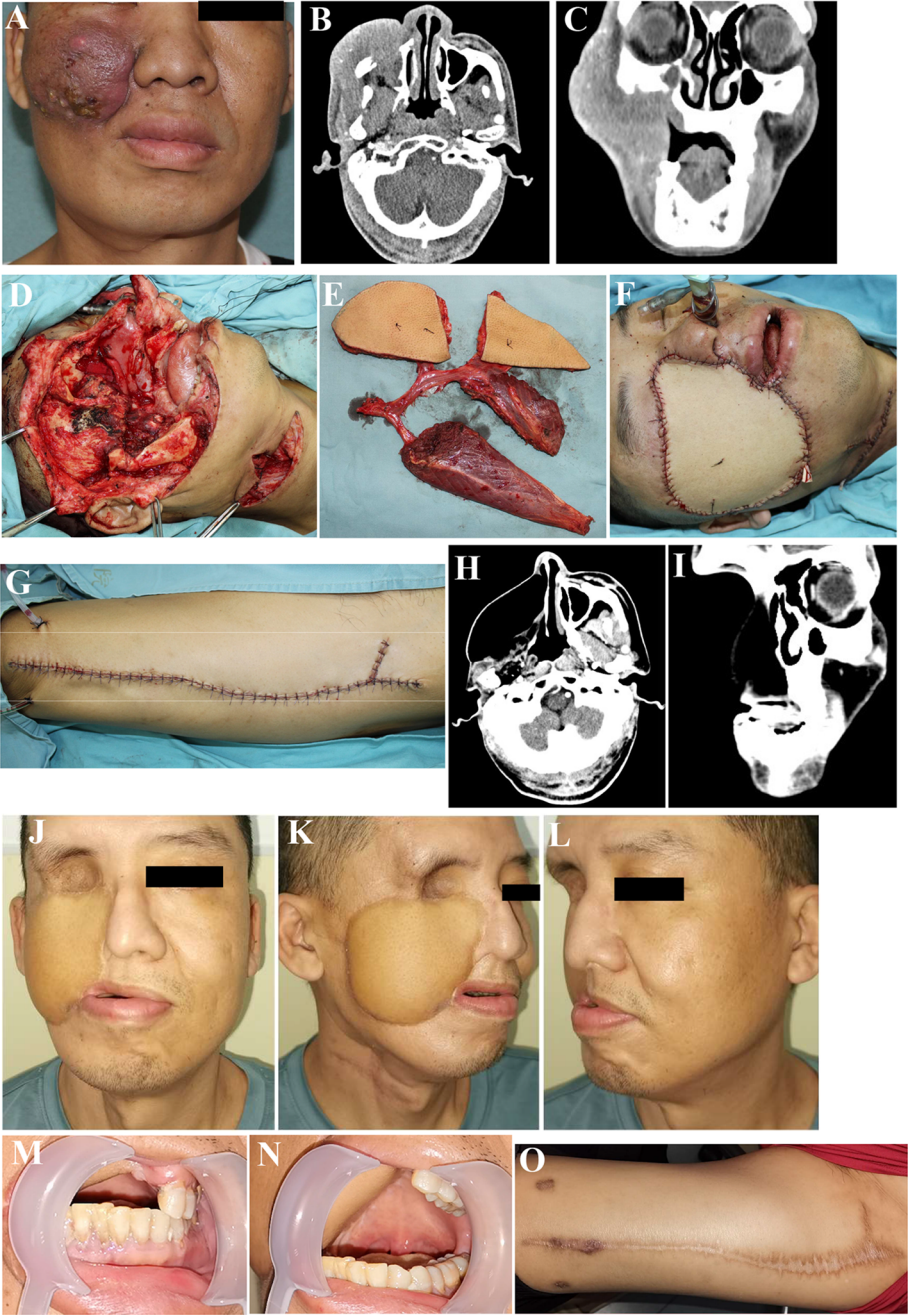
Reconstruction of complex lateral skull base defects using ALT chimeric flaps. (A) Recurrence of maxillary gingival squamous cell carcinoma, postoperatively after radiation therapy. (B, C) Preoperative CT. (D) Through-and-through cheek and lateral skull base defects after tumor resection. (E) Chimeric ALT, ALT, and rectus femoris flaps (entire rectus femoris). (F) Defects reconstruction using ALT chimeric flaps, separate flaps for intraoral mucosal and extraoral skin reconstruction, and rectus femoris flap for lateral skull base dead space filling. (G) Primary closure of the donor site. (H, I) Postoperative CT, 52 months postoperatively. (J–O). Sixty months postoperatively. ALT, anterolateral thigh.
